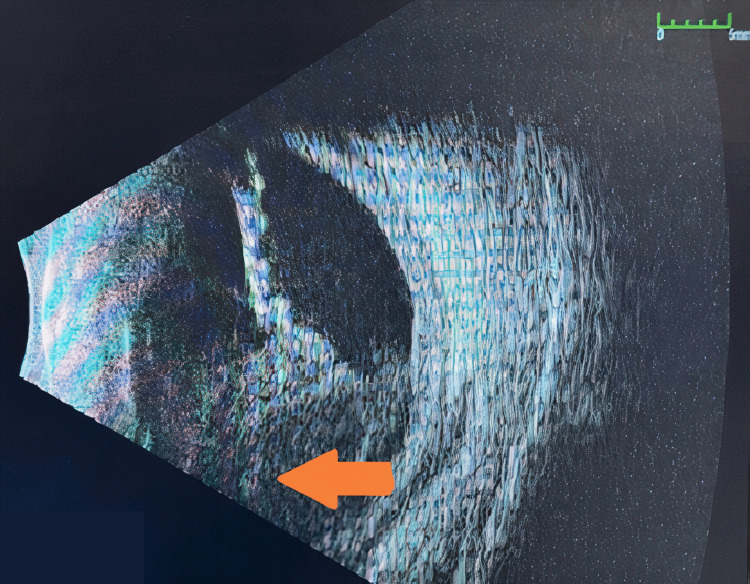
Right eye ocular ultrasound showing hyperechoic stranding in the vitreous cavity (orange arrow) along with serous choroidal detachment, suggestive of endogenous endophthalmitis.
